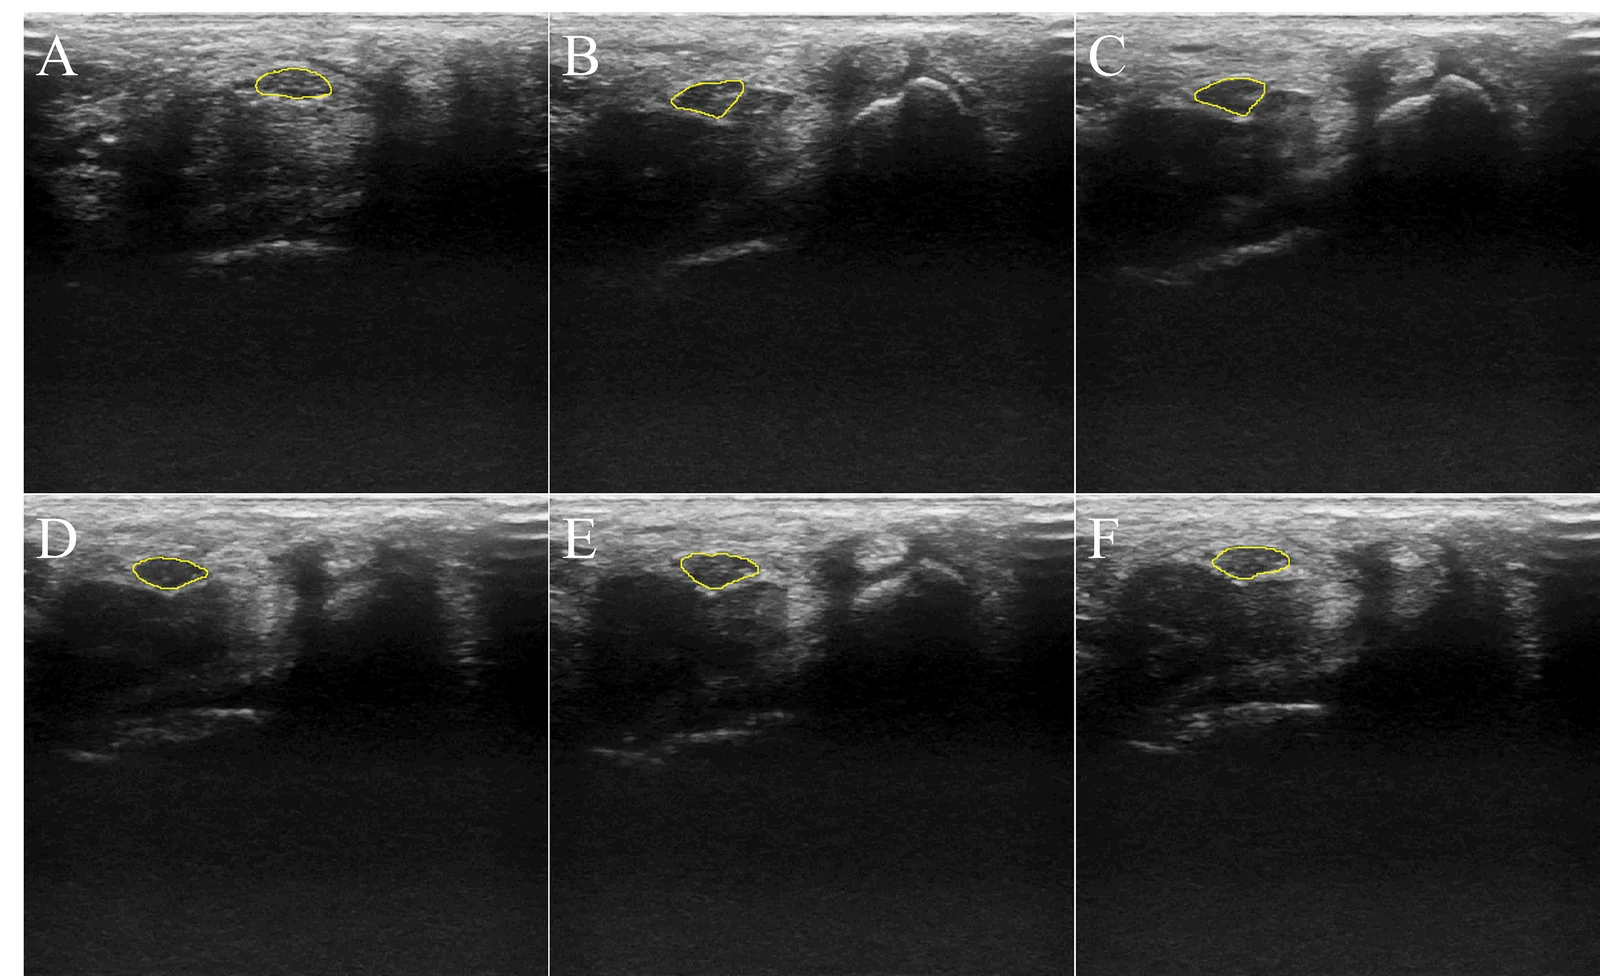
Representative ultrasound images of the median nerve under the EG condition (extension torque with grip) across the six sample points. (A) Baseline. (B) Torque maintenance. After torque release (C) 0.25 s. (D) 0.5 s. (E) 1.0 s. (F) 2.0 s. Yellow line was the outlined median nerve boundary used for MNCSA measurement.
